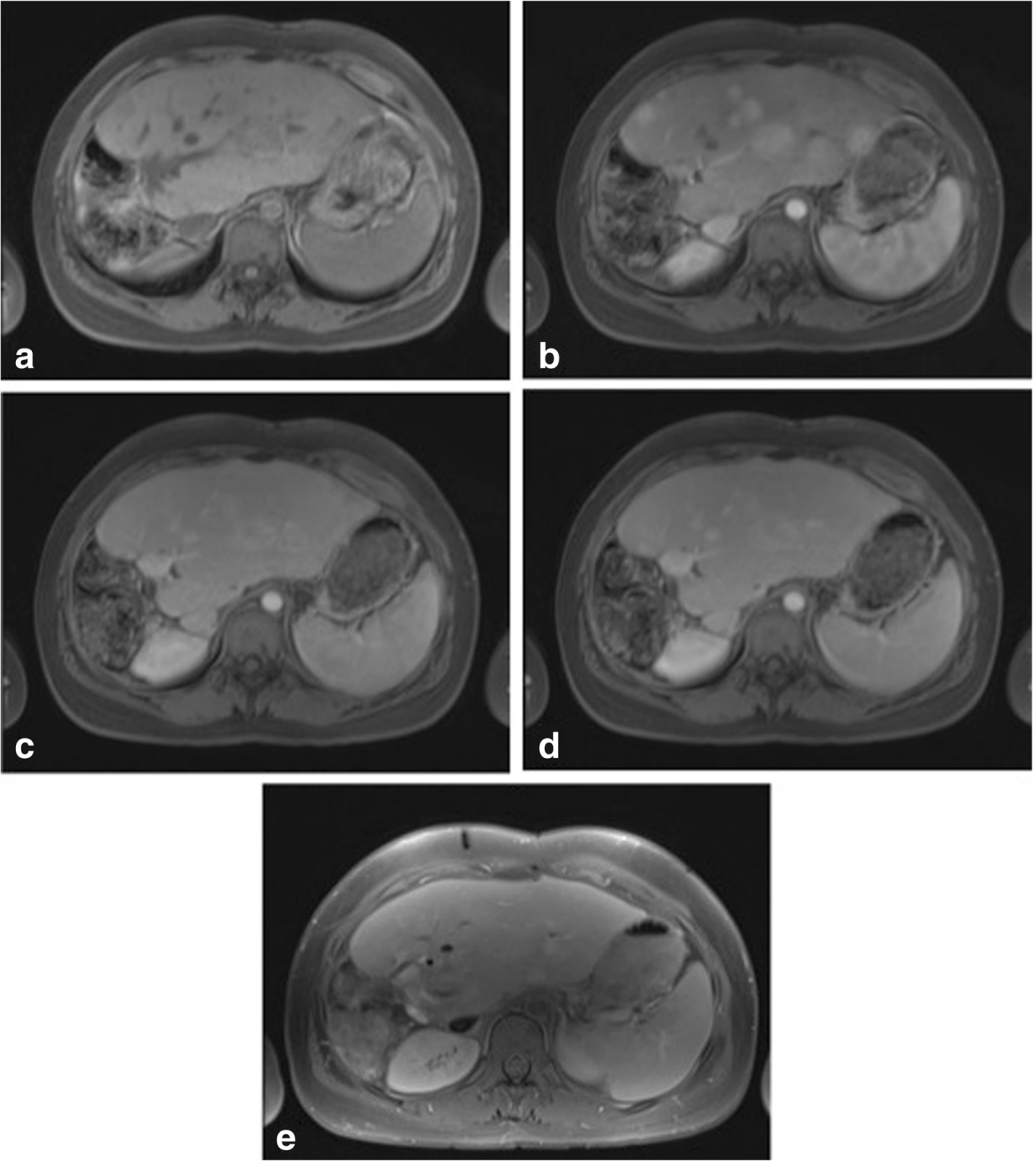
Multiple areas of focal nodular hyperplasia in a 17 year old patient with prior history of right hepatectomy for hepatoblastoma. Same patient as in Fig. 1. On the (a) pre-contrast T1-weighted fat-saturated images, the liver lesions are not visualized. Post gadobenate dimeglumine administration in (b) arterial phase imaging, there are multiple avidly enhancing lesions throughout the liver. These demonstrate similar internal signal intensity to the background liver parenchyma in (c) portal venous, (d) equilibrium and (e) 40 min delayed phases of imaging. There is no wash out of contrast material to suggest metastatic disease
